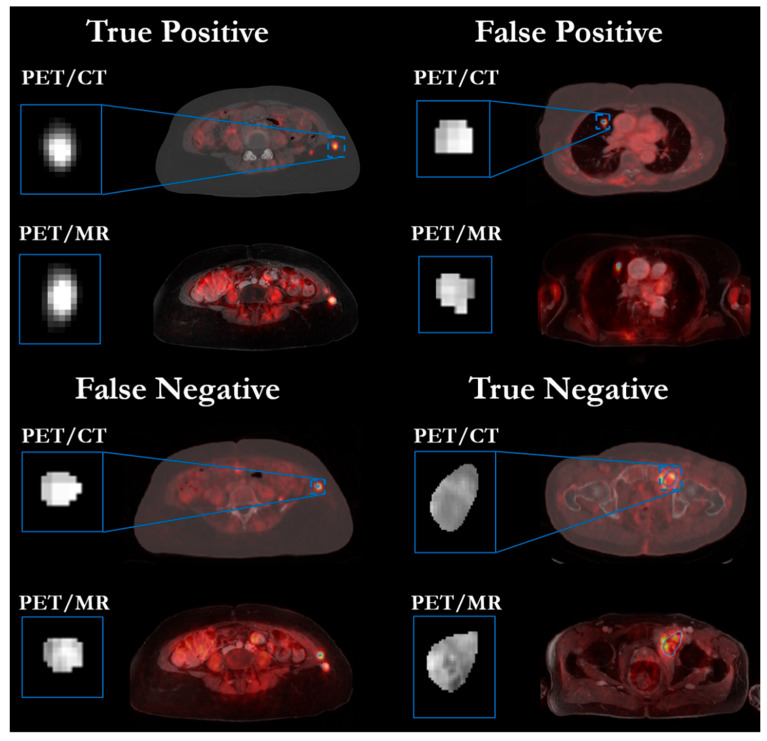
PET/MR and PET/CT images of four patients based on their CNN risk-classification output towards a true positive (true low risk), false positive (predicted low risk, but high-risk target), false negative (predicted high risk, but low-risk target) and true negative (true high risk). The PET images are color-coded overlaid to the CT/MR images. A zoomed version of the PET lesion is depicted to appreciate the textural inhomogeneity. The textural composition of the lesion guided the risk prediction.
